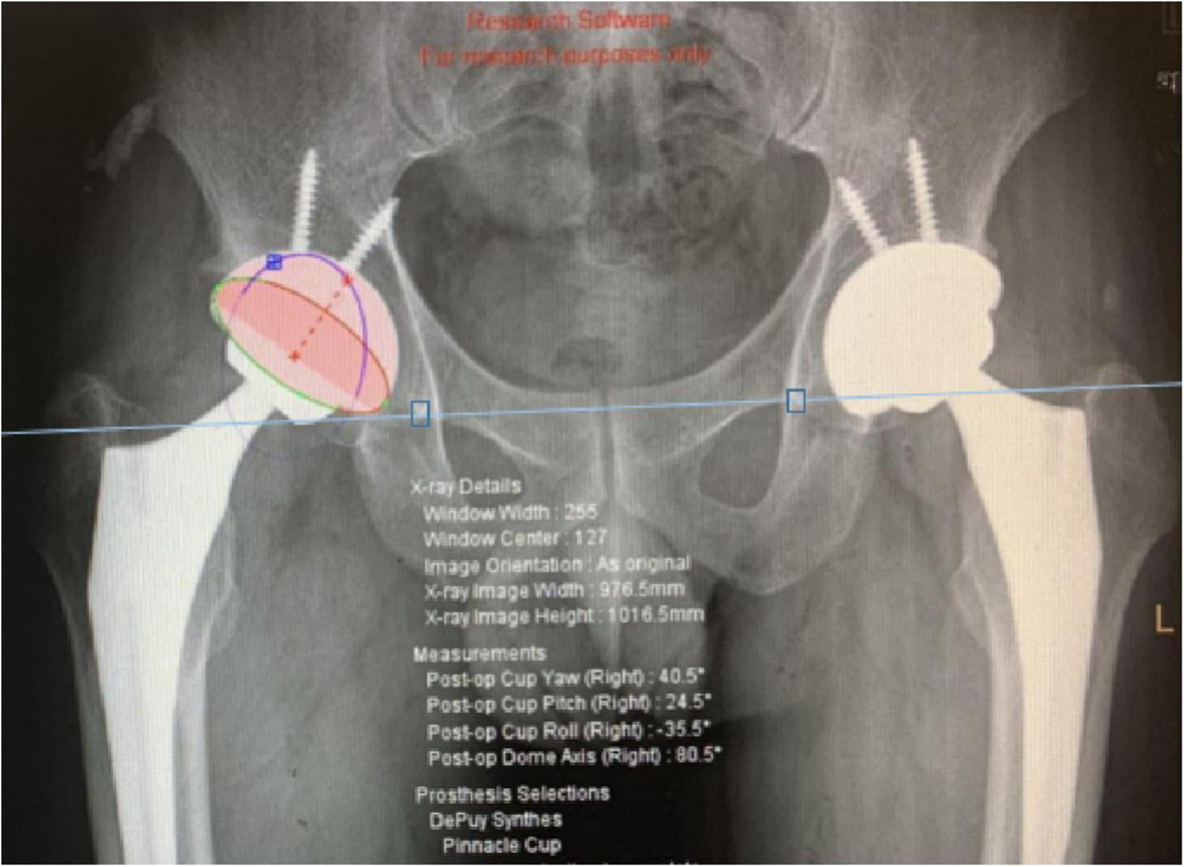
The method of measuring anteversion and inclination of acetabular cups on plain radiograph of pelvic with Orthoview Software
